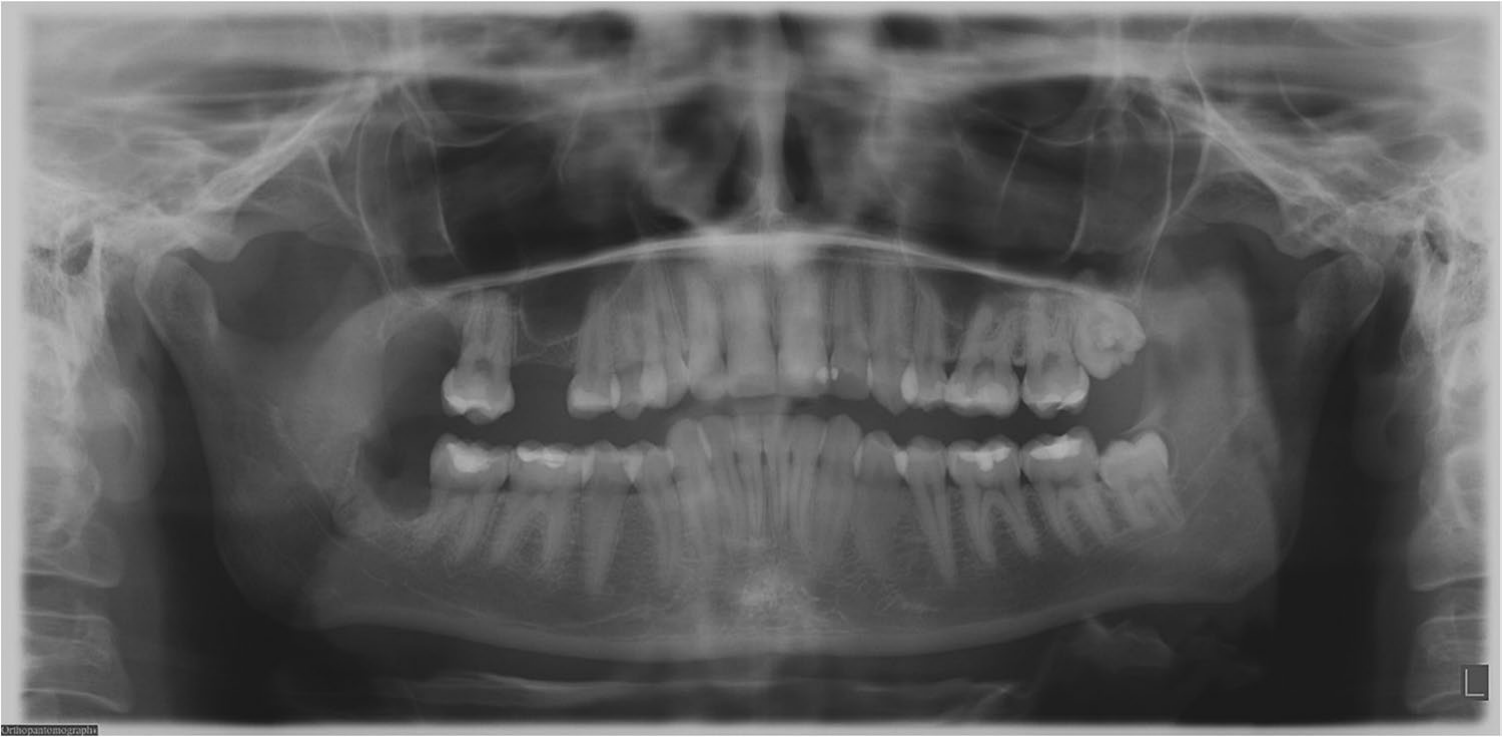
Panoramic radiograph the same patient, 2 months after coronectomy of the right mandibular third molar and removal of the right maxillary third molar
